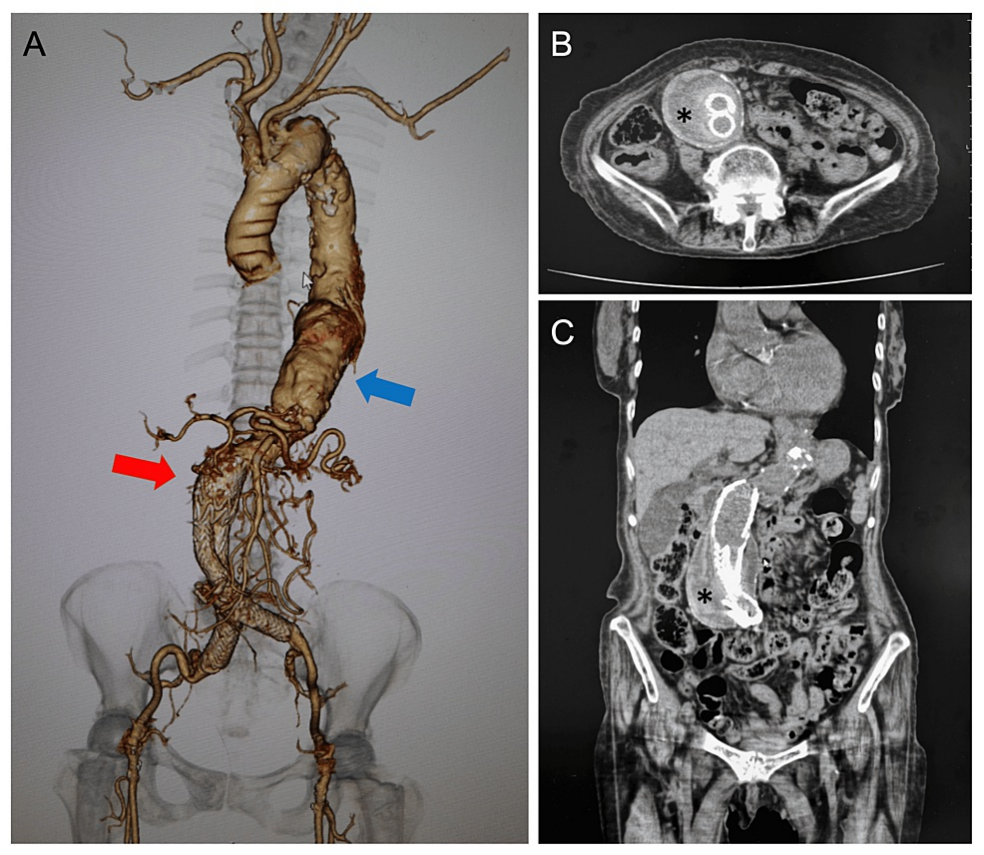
Computed tomography A: Contrast-enhanced computed tomography (CT) reconstruction image. Untreated thoracic aortic aneurysm, 54mm diameter (blue arrow), and abdominal aortic aneurysm after stent graft insertion (red arrow). B: CT cross-sectional image, C: CT coronal section image. Abdominal aortic aneurysm after stent graft insertion, 60 mm diameter aneurysmal sac (asterisk).
